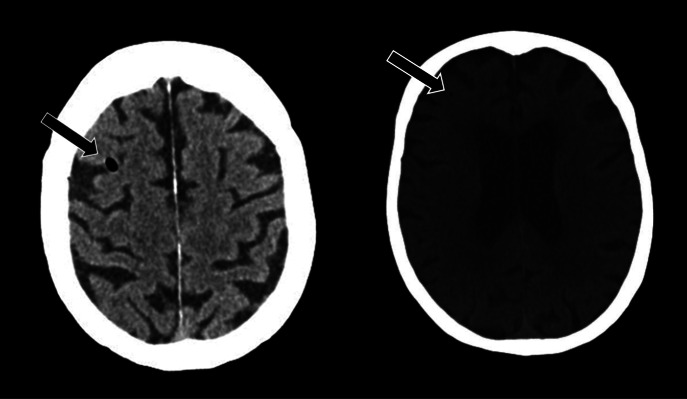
Computed tomography head without contrast demonstrating air embolisms (arrow).
